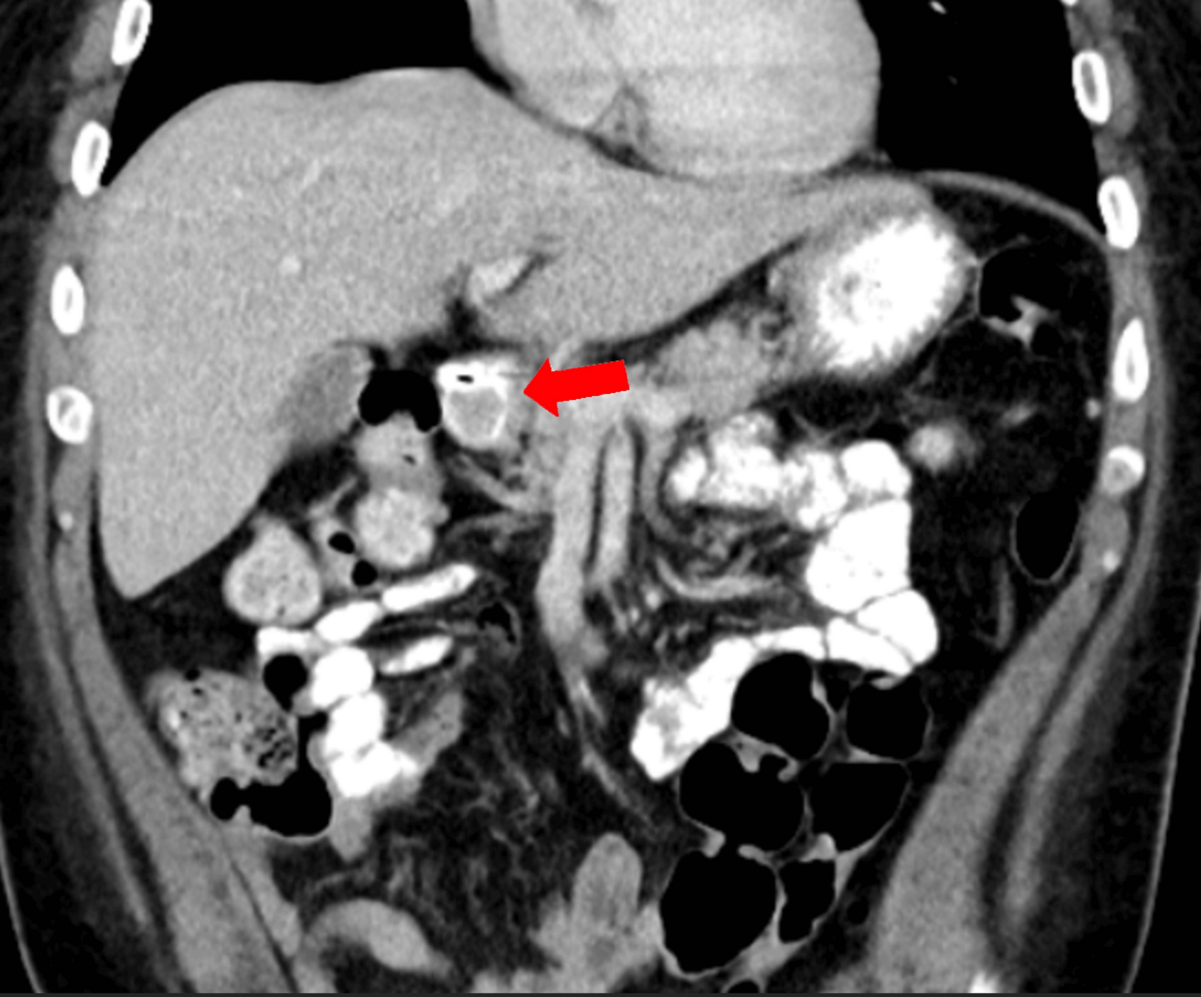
Computed tomography with oral and intravenous contrast showing duodenal mass in coronal view without evidence of locoregional invasion
